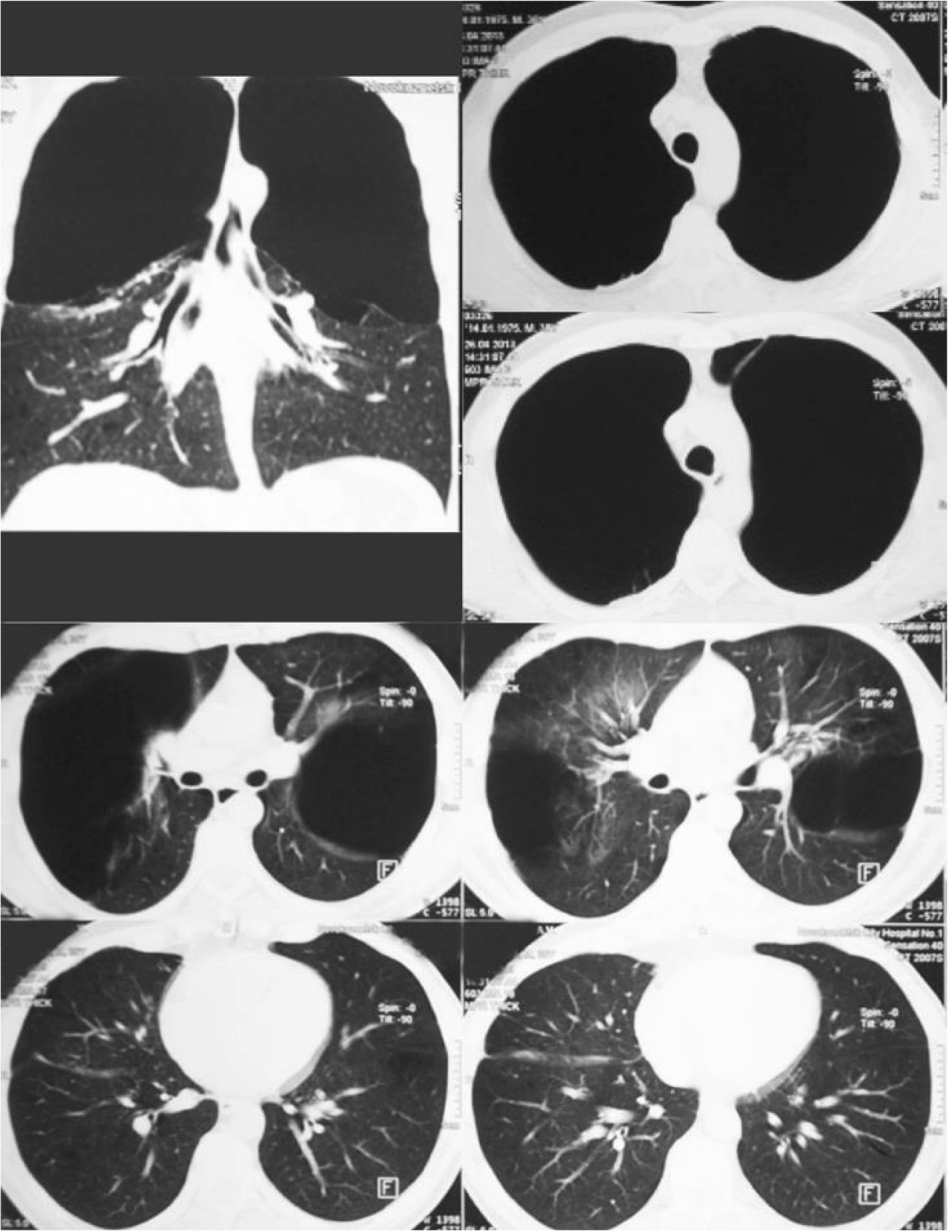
Chest CT scan on admission. Upper sections of the both lungs contains gigantic bullae over ½ of hemithorax volume. Pulmonary parenchyma in middle and lower sections is preserved, but emphysemateus. It contains singular bullae
